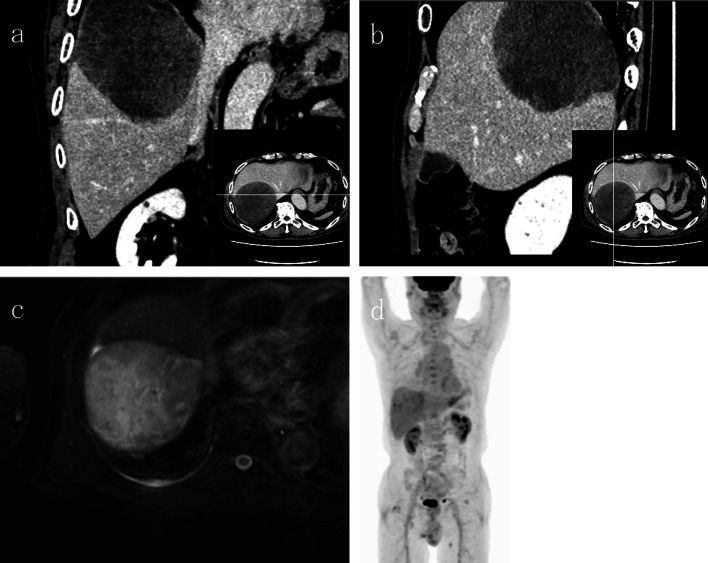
Contrast-enhanced computed tomography (CT), contrast-enhanced magnetic resonance imaging (MRI), and positron emission tomography (PET). a, b CT shows a well-circumscribed and smooth-marginated, huge 10-cm mass under the right diaphragm. The main feeder for the tumor is the right inferior phrenic artery. c MRI demonstrates heterogeneous high signal intensity on T2WI. d PET revealed abnormal uptake in the tumor with a SUVmax of 4.6
